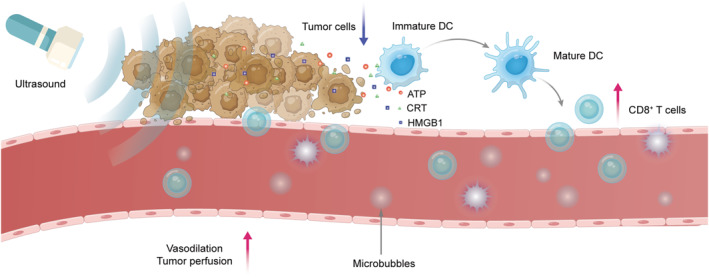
Mechanisms of non‐thermal ultrasound‐enhanced sono‐immunotherapy.
